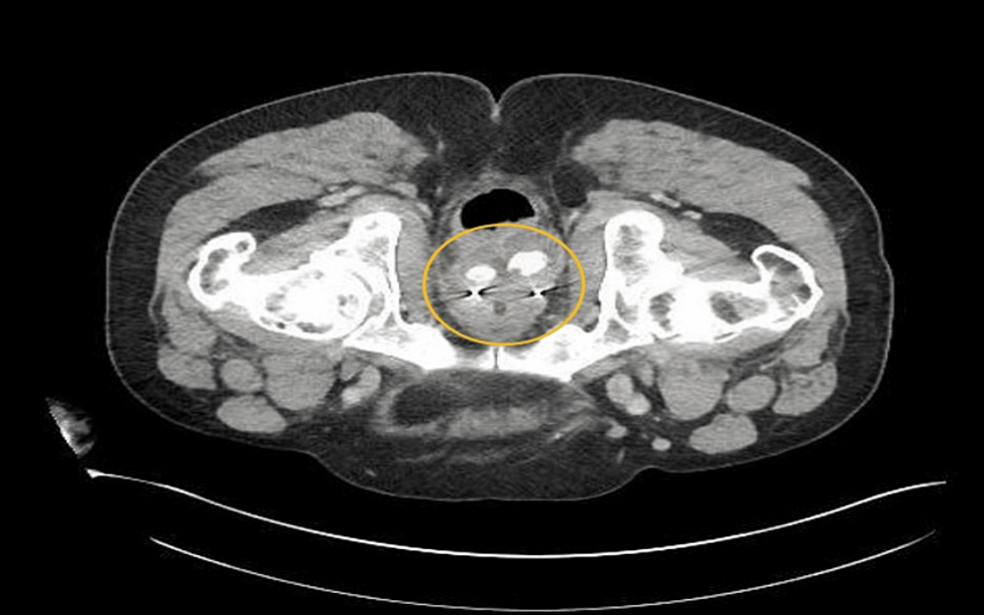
CT of the pelvis with contrast (axial view) Rim-enhancing collection (shown within the yellow circle) measuring 5.7 x 3.5 x 5.4 cm with internal areas of high attenuation between the prostate and rectum correlating with the location of SpaceOAR hydrogel placement with increased extension inferiorly into the perineum.
